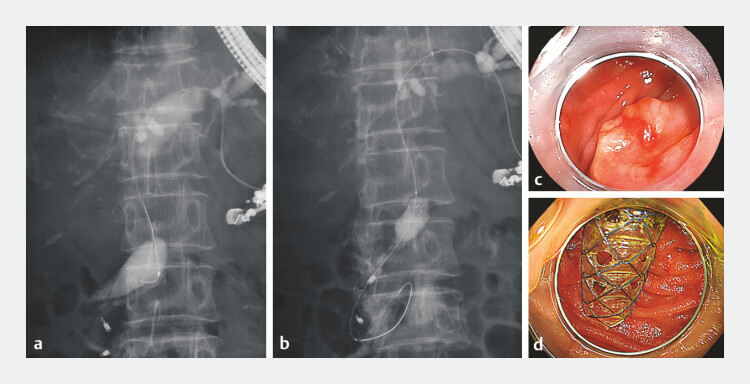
A fluoroscopic image of interventional endoscopic ultrasound, along with endoscopic images before and after creating the gateway.
